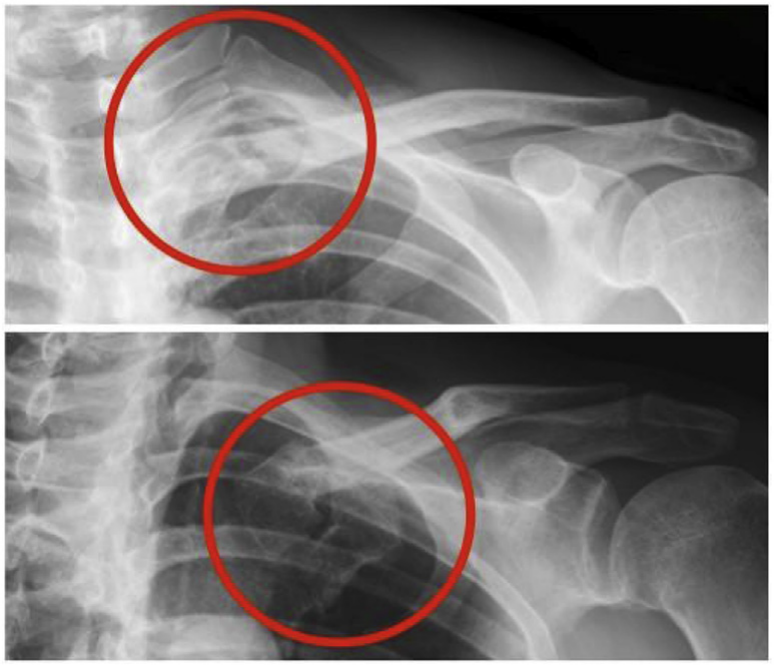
Preoperative radiographs of the left-sided medial clavicle fracture. The red circles indicate the fracture site.
